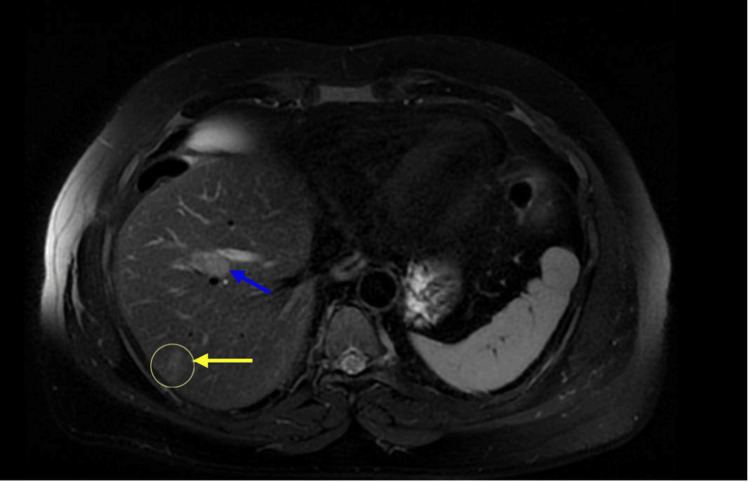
Abdominal MRI showing multiple liver metastatic lesions: blue arrow showing a lesion on the right pedicle and yellow arrow showing a lesion in segment 6 MRI: magnetic resonance imaging
